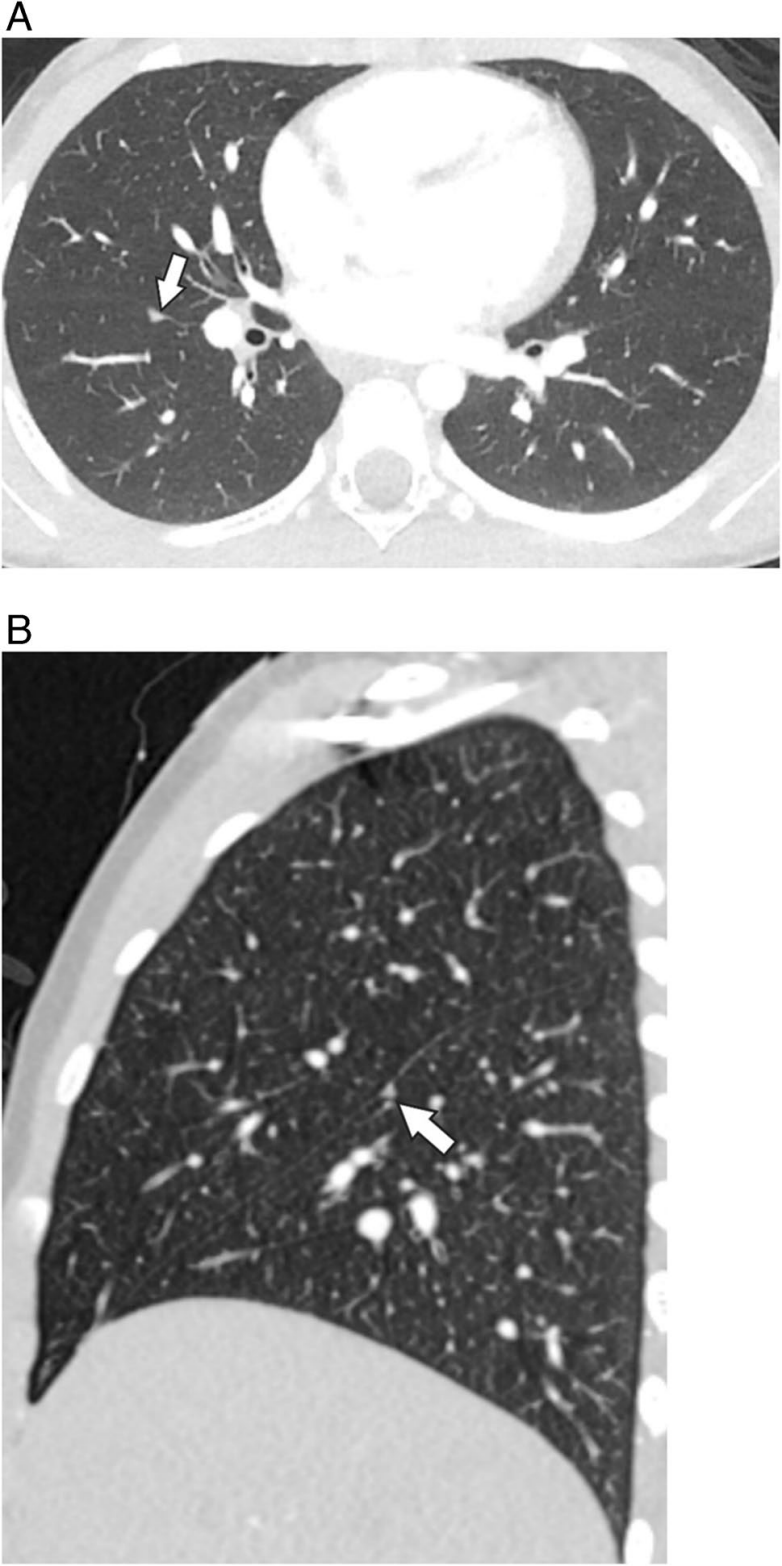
Benign perifissural lymph node in a 9-year-old female who presented with chest pain after gymnastics injury. A Axial and (B) sagittal contrast enhanced CT show an incidental 3 mm triangular, solid pulmonary nodule abutting the right major fissure that is most consistent with a lymph node (*arrows*)
